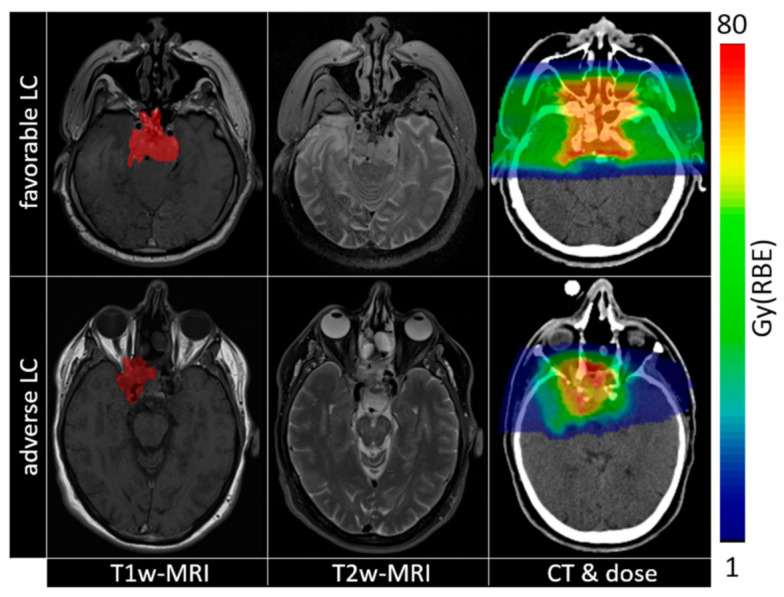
Imaging (from left to right: T1w-MRI, T2w-MRI, CT) and dose maps (overlaid to CT) for patients with opposite local control evaluation (top row favourable LC, bottom row adverse LC). Tumour contours are shown as red overlays on T1w-MRI images.
